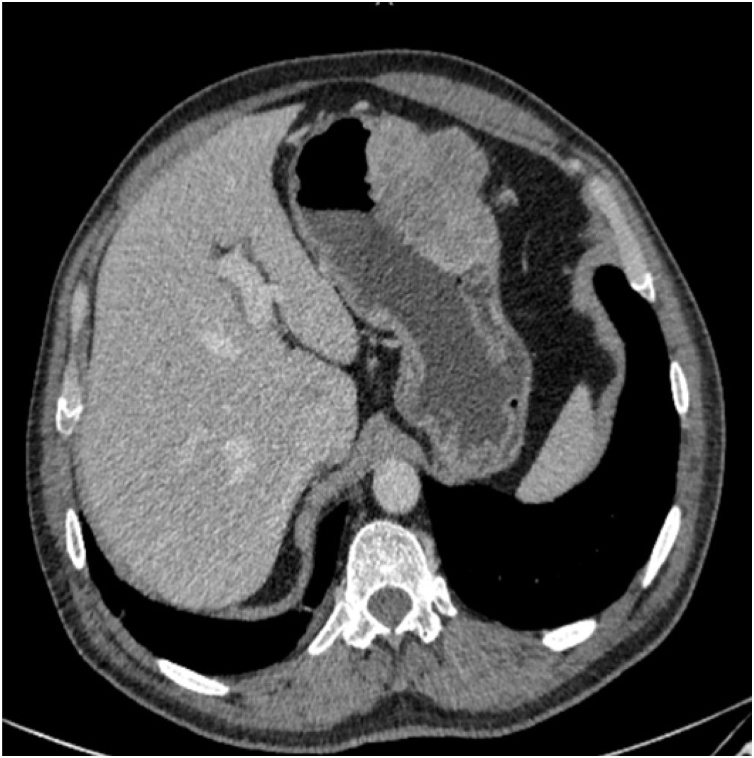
An axial plane of CT abdomen showing large lobulated mass at the greater curvature.
